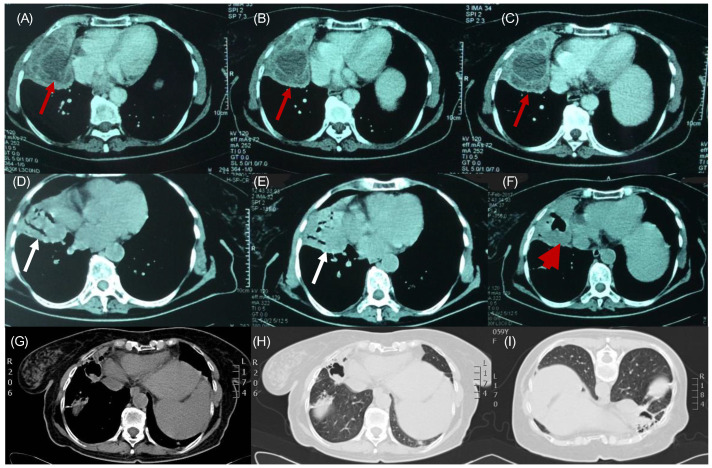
1 year prior:* (A–C): Multiple unraptured hydatid cysts, containing daughter cysts. The fluid inside the cysts has a water density (red arrows). During hospital stay: (D,E): Combo or onion peel sign. We see air–fluid levels in the endocyst and pericyst (white arrows). (F): Water-lily sign or camalote sign. Complete rapture of the endocyst. Membranes float in the remaining fluid. The last two signs indicate complete rapture (red arrowhead). Follow-up: (G) Thorax CT 6 months after treatment: Intracancerated membrane sign or mass in the cavity sign on the mediastinal window, (H) on the lung-on-lung window, and (I) at prone position, the remaining solid components wrinkle to the dependent wall of the cyst.
